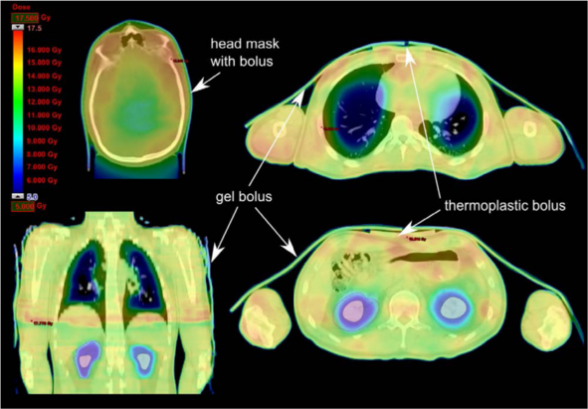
Different CT slices of the cranial part of patient H.L. The *arrows* indicate the positions of the thermoplastic and gel boluses. The colour wash presentation of the dose distribution shows the dose reductions of up to 50 % in the lung and the kidneys. In patient H.L. the kidneys were spared due to pre-TBI renal insufficiency
